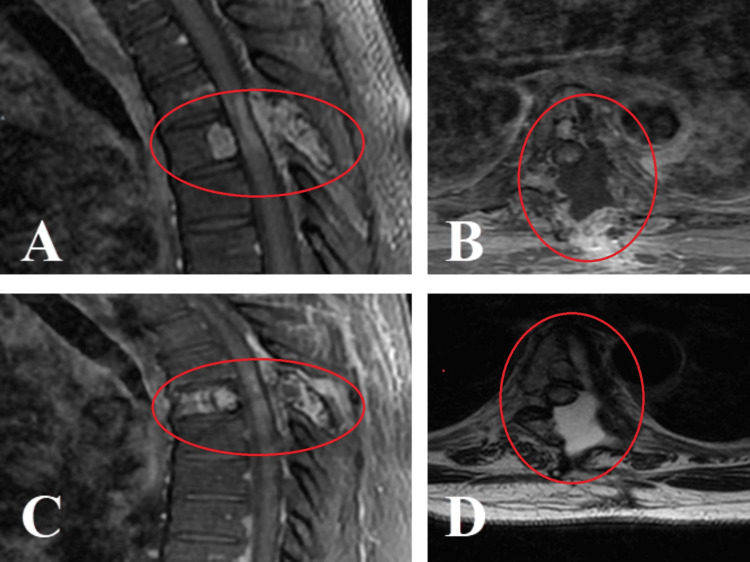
Post-operative MRI scan (A and B) shows a hypointense area that corresponds to the third project of circumferential decompression performed using MIS. Post-radiotherapy MRI scan (C and D) shows improvement in the width of the spinal canal and resolution of spinal cord compression. MIS: Minimally Invasive Surgery.
